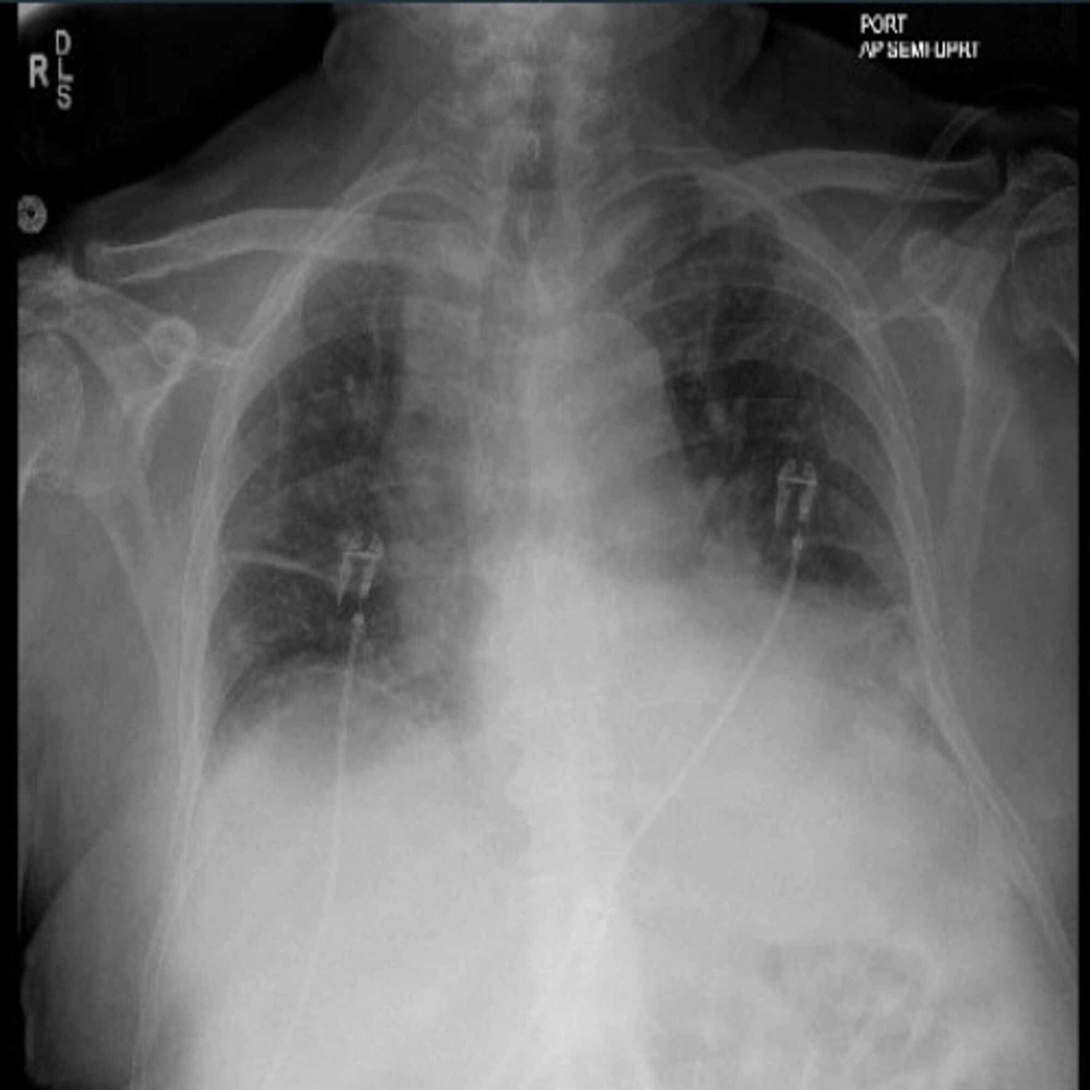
Anteroposterior chest X-ray showing findings suggestive of mild bilateral pleural effusion
